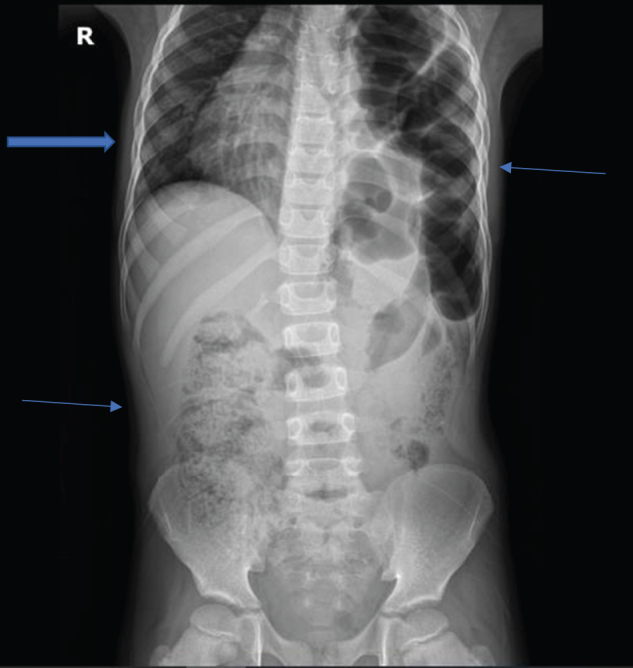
Chest-abdominal X-ray erect view showing loops of large bowel in the left hemithorax with loss of left hemithorax dome (thin blue arrow on the upper left side), fecal material in the ascending colon (thin blue arrow on the lower right side), and mediastinal shift to the right with pseudodextrocardia (thick blue block arrow on the upper right side).
